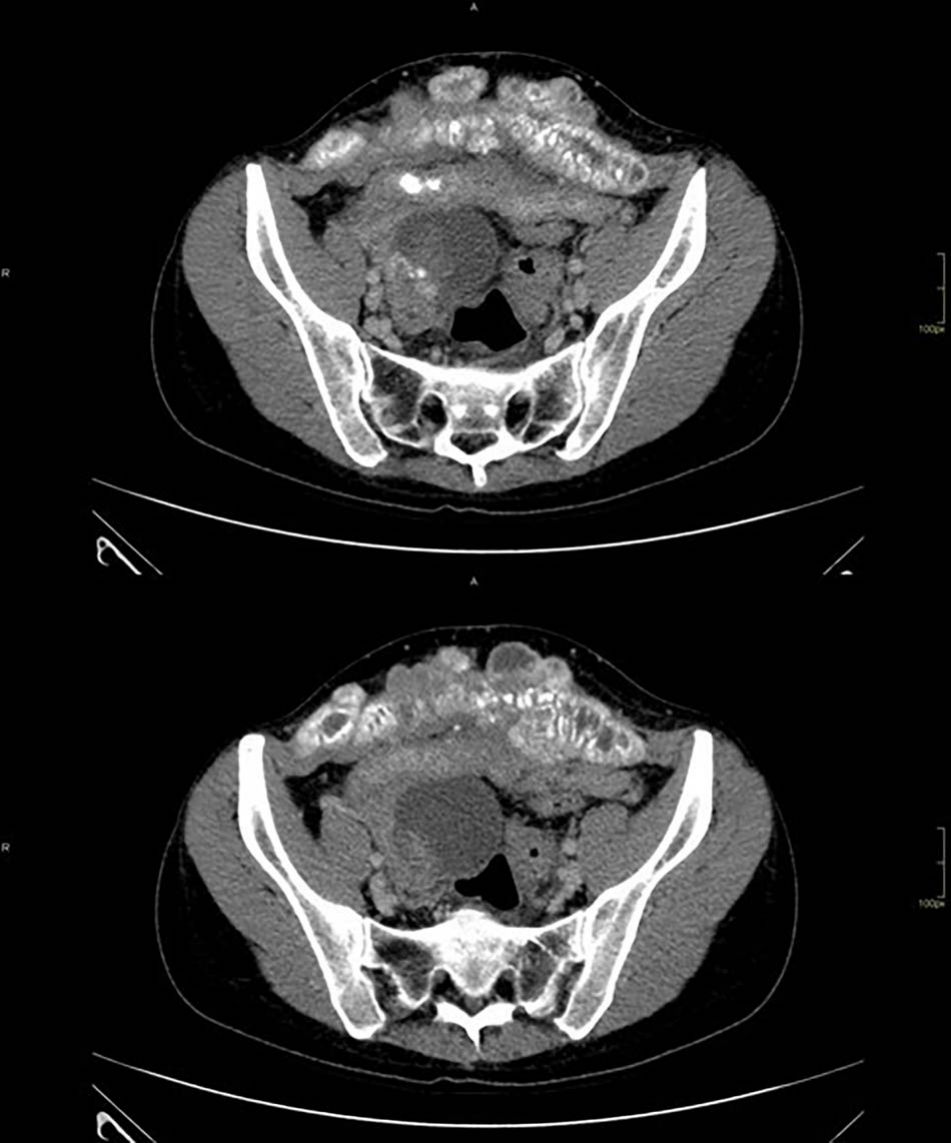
CT with heterogeneous solid-cystic lesions with contrast enhancement, septations and gross calcifications, in the topography of the uterine attachments, measuring 5.8 × 4.9 × 5.0 cm on the right (74 cm^3^ volume), 7.8 × 8.7 × 7.9 cm (278 cm^3^ volume) in the region of the rectouterine pouch and 2.9 × 2.4 × 2.3 cm (8.3 cm^3^ volume) in the left adnexal region.
